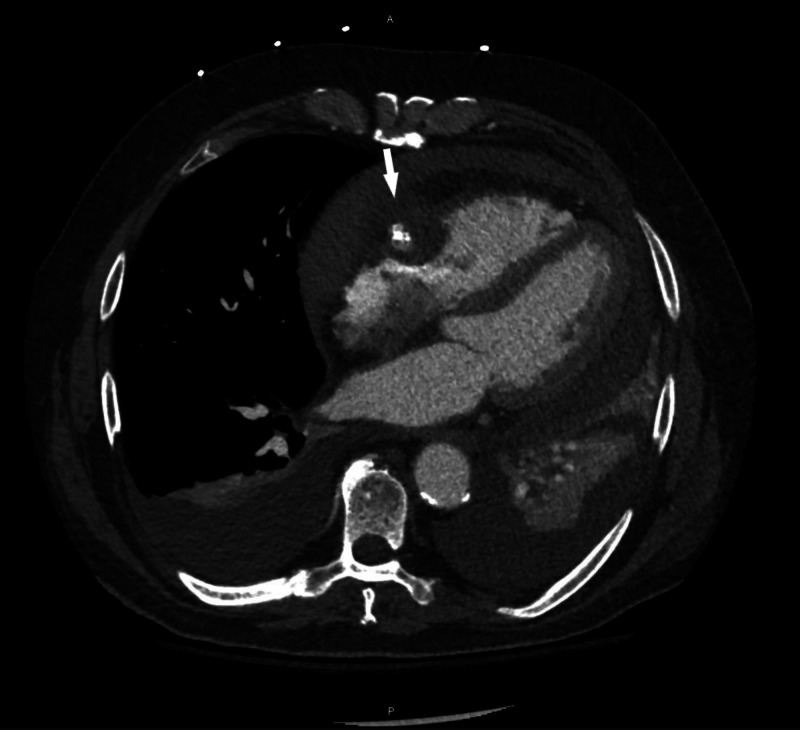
CT angiography of the thorax, axial view High-density pericardial effusion consistent with hemopericardium. High-density fluid can also be observed surrounding the right coronary artery, consistent with aneurysm (arrow). Bilateral pleural effusion can be seen.
